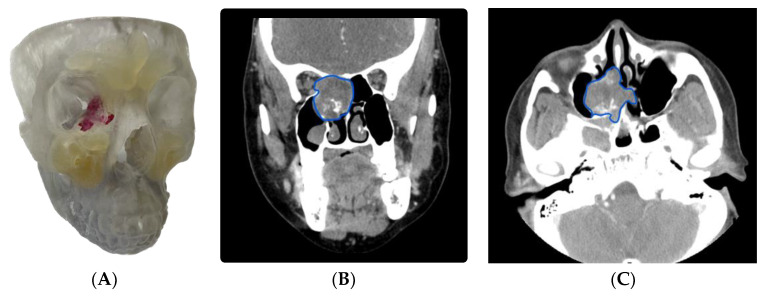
(A) 3DM rendering of the tumor and related bony and vascular structures, (B) coronal CT highlighting the complexity of the tumor location (blue outline highlighting tumor), and (C) axial CT image highlighting the tumor’s relationship to the petroclival internal carotid artery.
